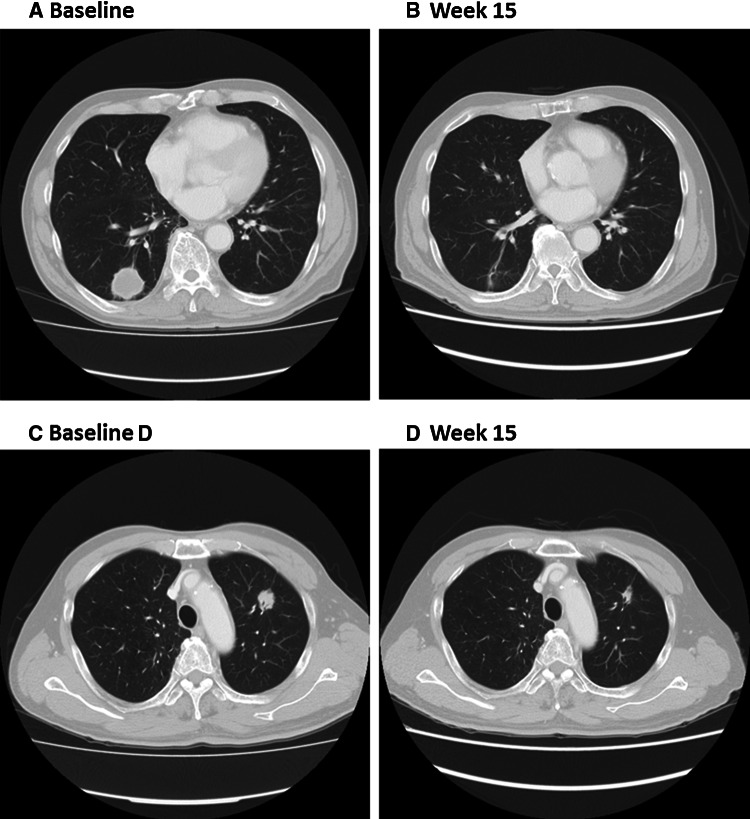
Partial response to veliparib 40 mg BID and carboplatin and paclitaxel. CT images at baseline (a, c) and following treatment (15 weeks; b, d) in an approximately 70-year-old male with advanced NSCLC
